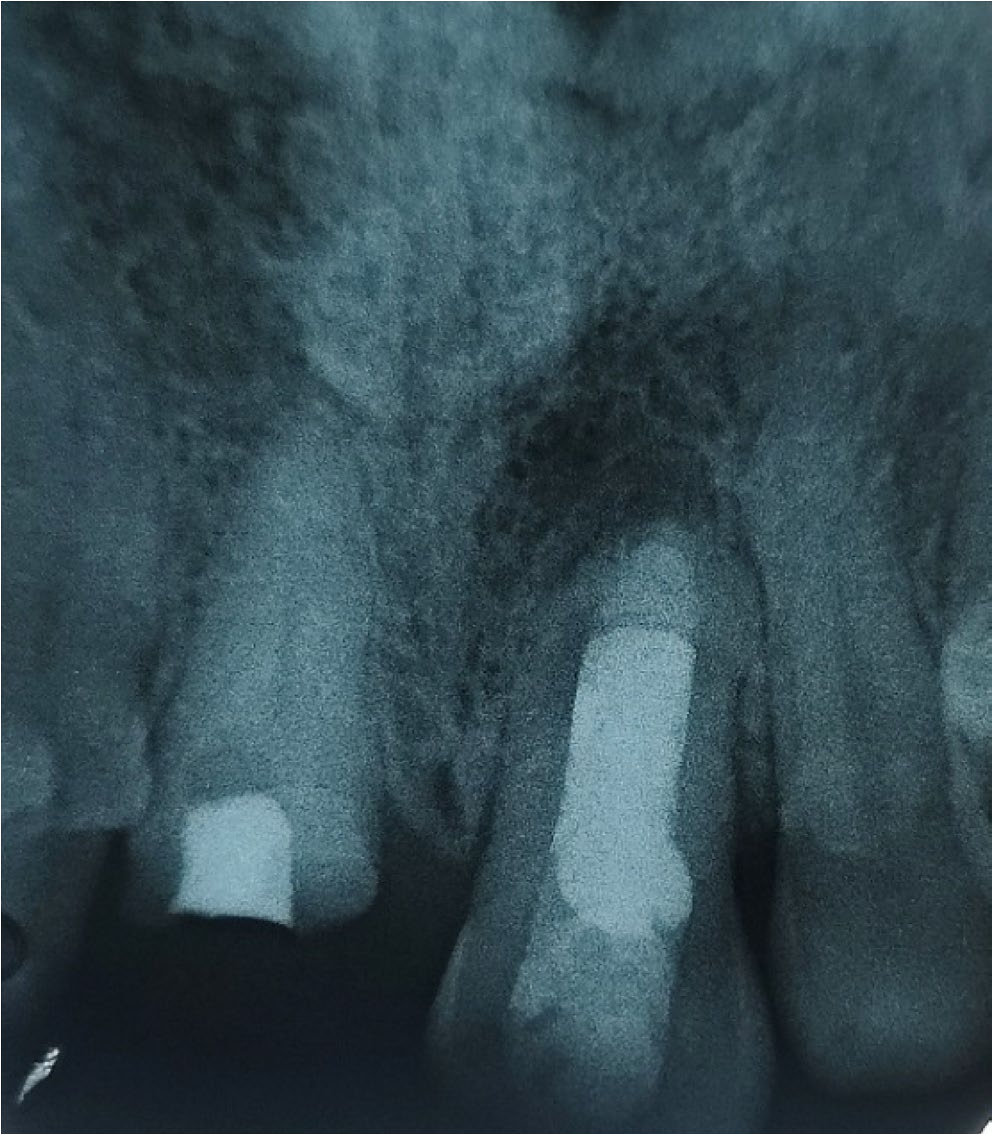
Pre‐operative radiograph showing presence of apical radiolucency.
